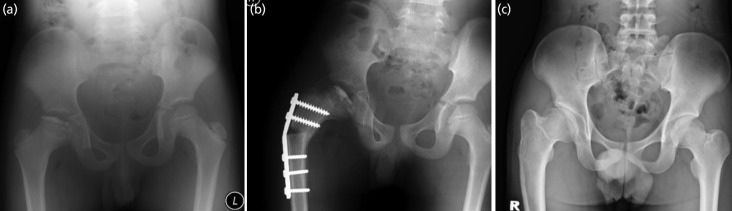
The series of radiographs belongs to patient no.1. (a) The presentation at the age of 9 showed a fragmentation phase with Herring C. (b) The immediate post-surgery showed good containment. (c) The hip at the age of 19 showed Stulberg III.
